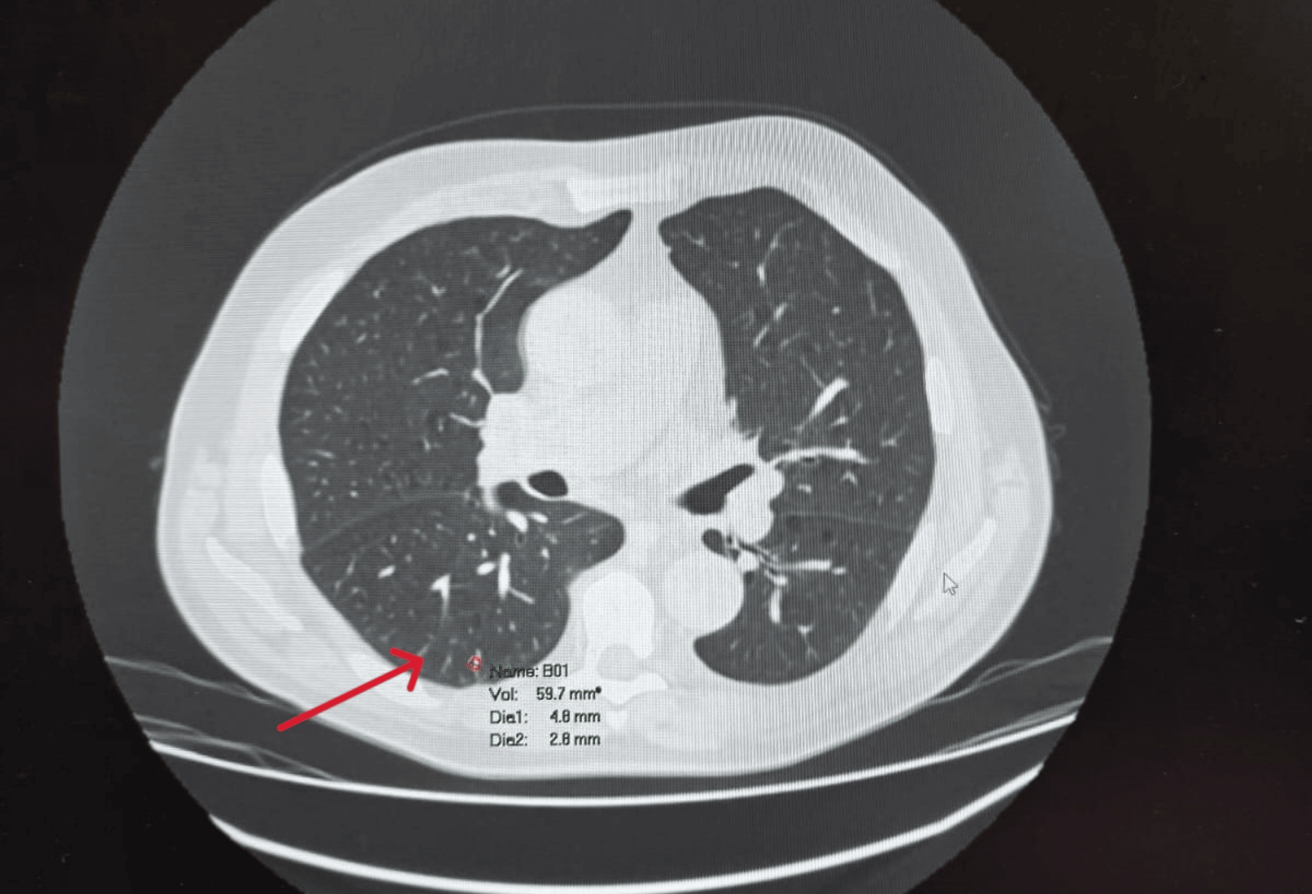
CT thorax CT thorax showing approximately 5mm lung nodule (Red Arrow) in the posterior aspect of right lower lobe.
